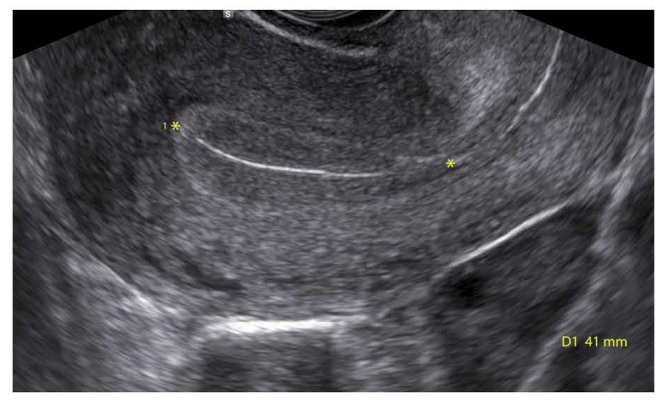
Evaluation of endometrial length of uterus by vaginal ultrasound.
